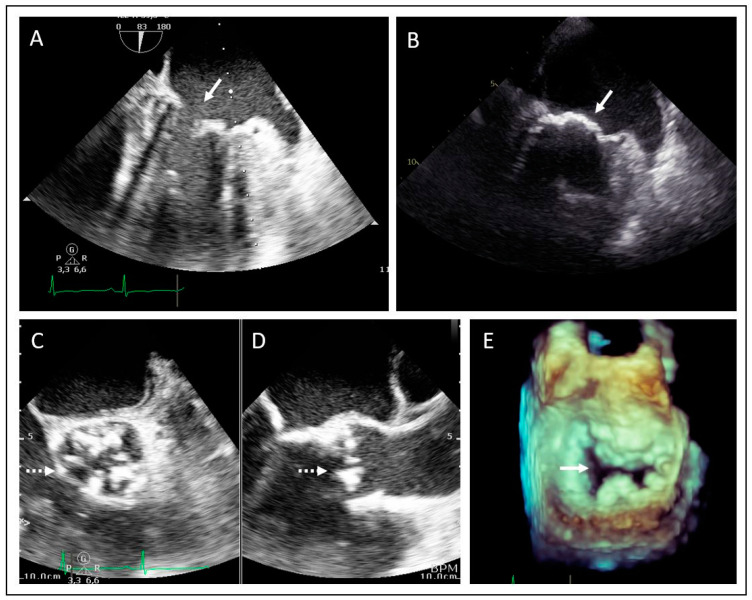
Transesophageal echocardiographic examination of the proband of family 2 showing aortic valve stenosis and mitral valve stenosis. (A,B) Mid-esophageal 2-chamber view demonstrating severe mitral valve calcification (arrow). (C) Mid-esophageal aortic valve short axis view and (D) long axis view, showing severe aortic calcification (dotted arrow). (E) 3D dataset of the mitral valve during diastole showing significant calcification and stenosis (arrow).
